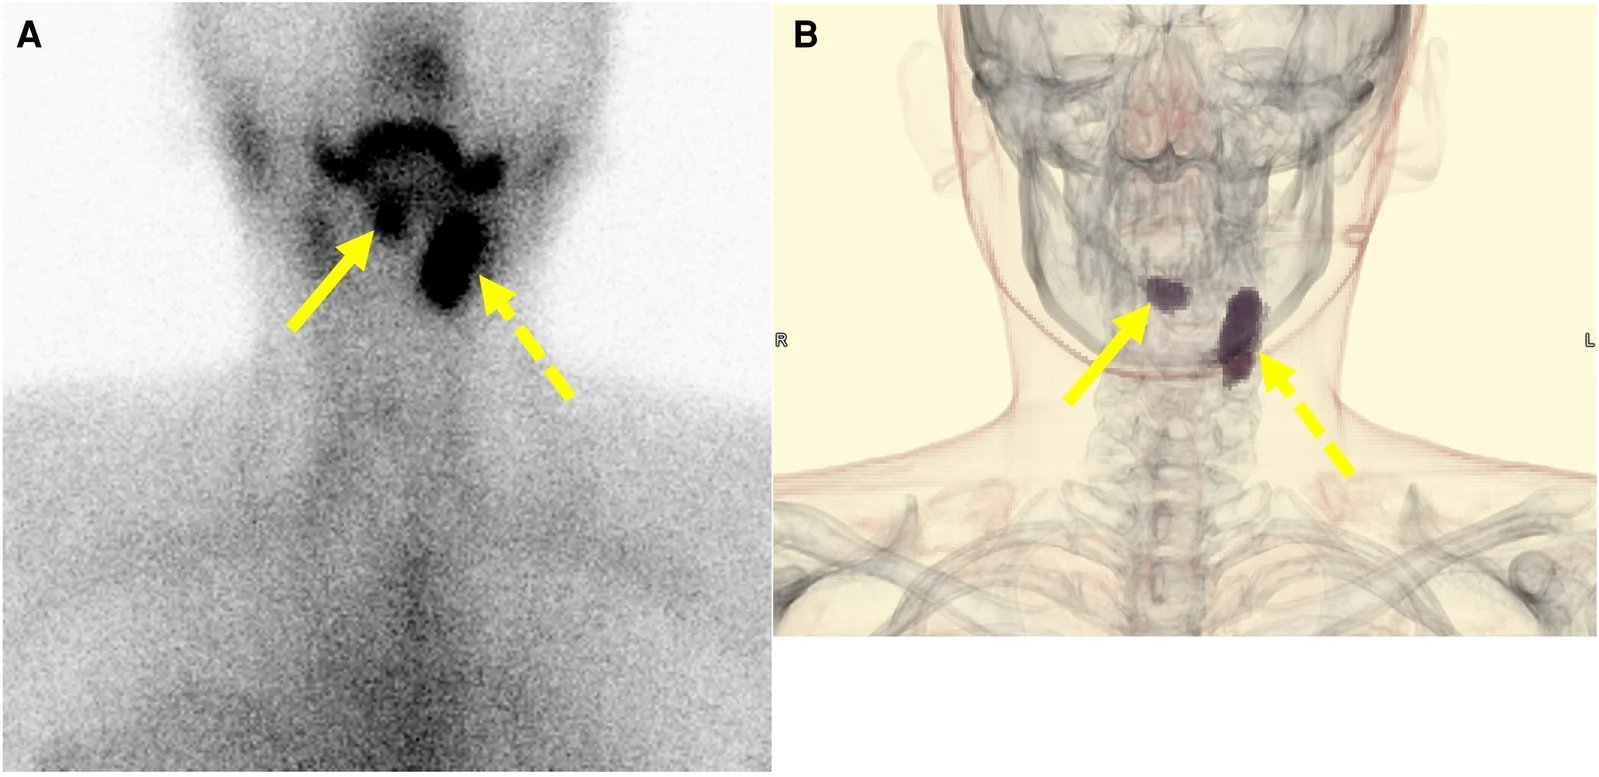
Images of ^99m^Tc thyroid scintigraphy and SPECT-CT. (A) Tracer accumulation is located at the base of the tongue and at the left parapharyngeal space. (B) 3D construction of SPECT-CT shows mass images at the same two locations. Abbreviations: SPECT-CT, single-photon emission computerized tomography with computed tomography.
